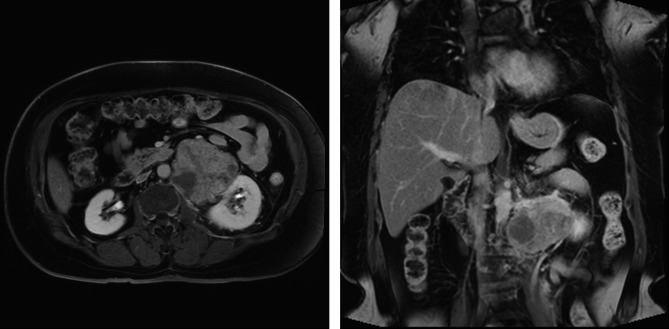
The MRI showed a heterogeneous left retroperitoneal tumor
